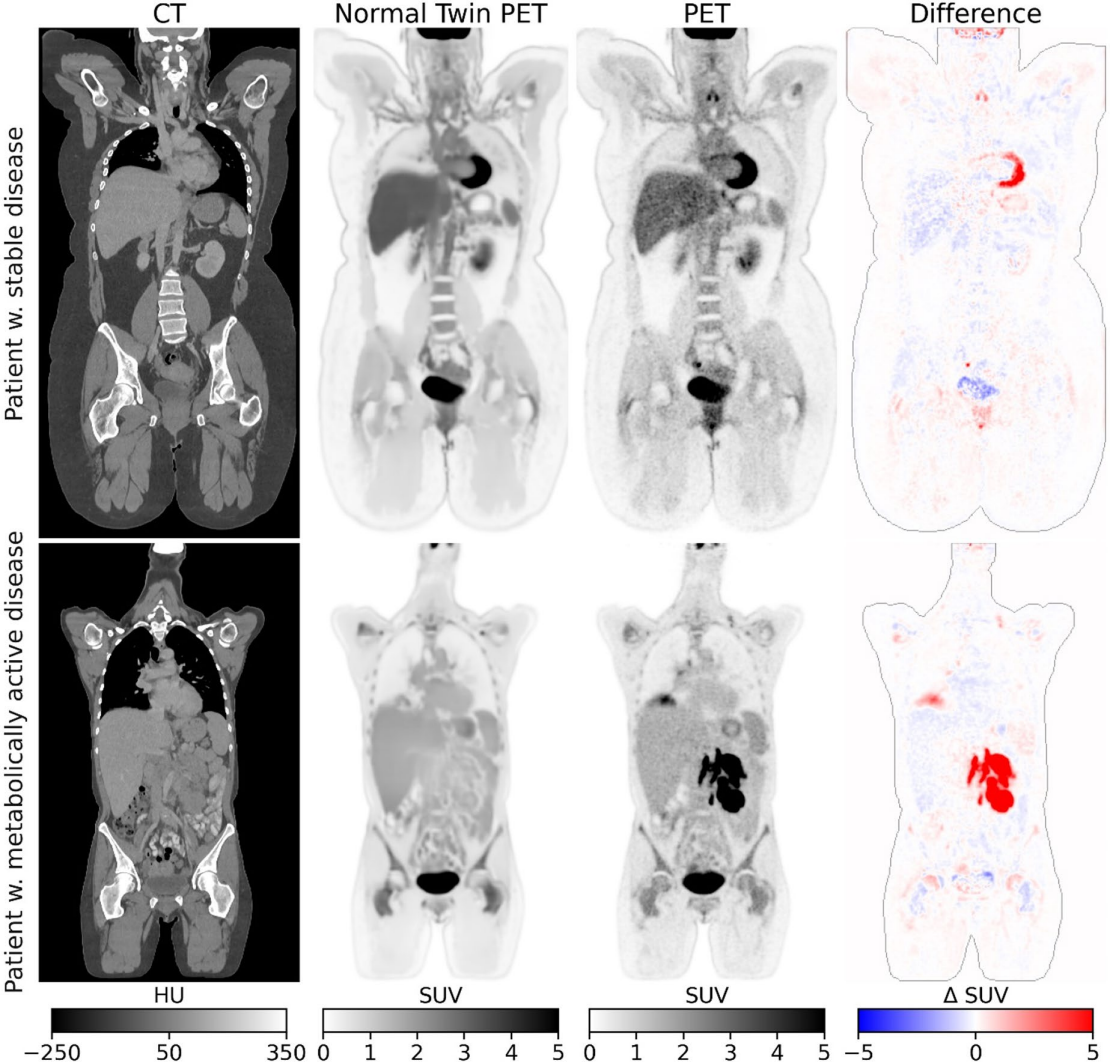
Two characteristic PET/CT test studies from the stable (top) and metabolically active (bottom) lymphoma cohorts, showing the corresponding normal twin PET images and the difference between true and normal twin PET images. Normal FDG variations are captured by the ntPET, resulting in near-zero differences in normal regions and large differences in malignant regions.
